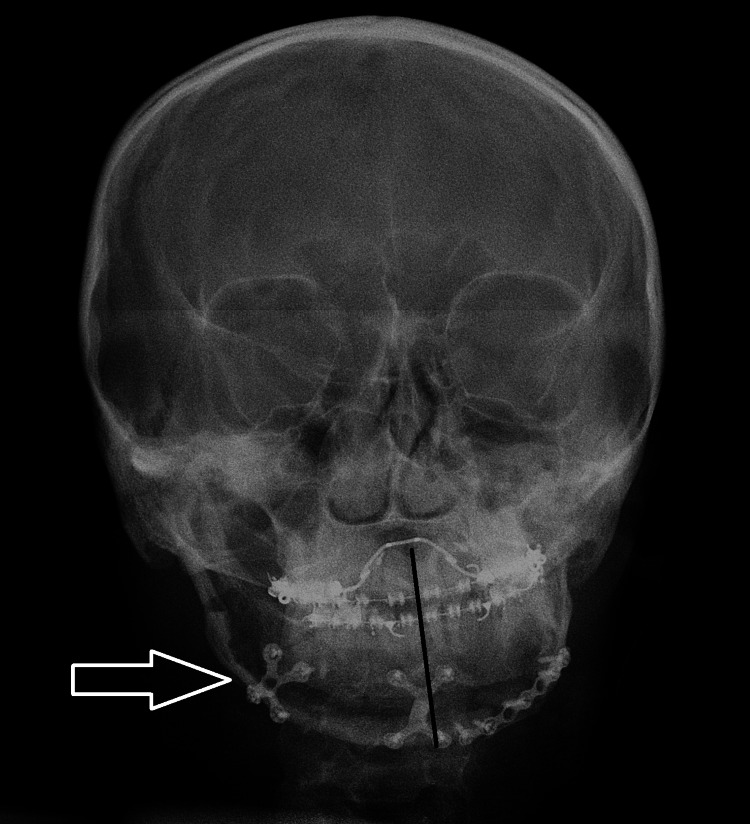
Immediate postoperative skull X-ray (posteroanterior view) showing correction in midline along with contour of the mandible (arrow).
